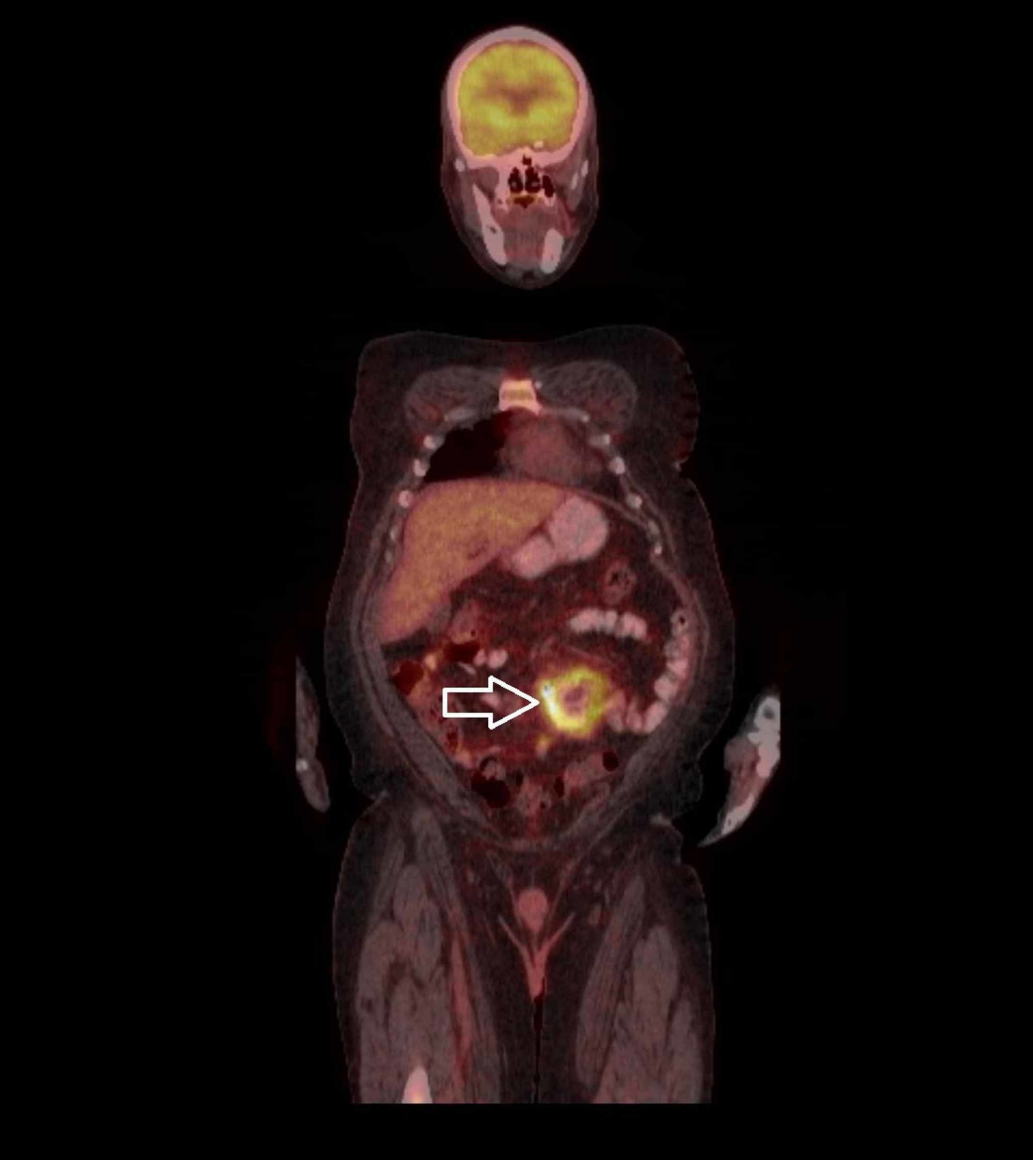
Whole body PET-CT PET-CT, positron emission tomography-computed tomography
